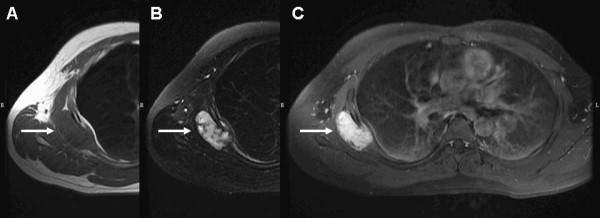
Magnetic resonance imaging of chest shows a 5.2×4.5×4.6cm mass involving the right serratus anterior muscle, the right fifth intercostal space and the subpleural space (white arrow). (A) T1-weighted image shows isointense to hypointense relative to the muscle. (B) T2-weighted image shows heterogeneously hyperintense with some low-signal spots. (C) T2-weighted image with gadolinium injection shows inhomogeneous contrast enhancement.
